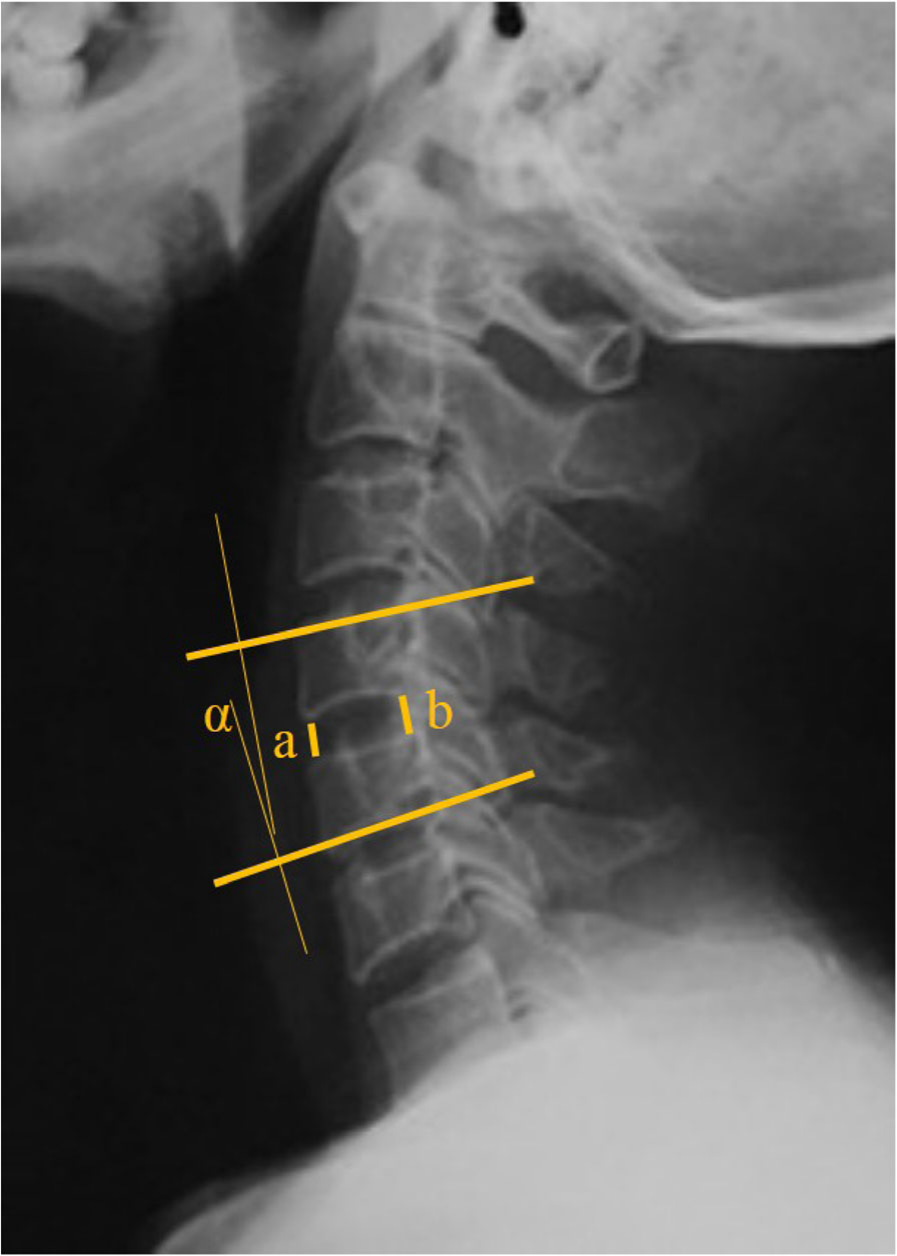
The functional spinal unit angle (*α*) was examined on lateral radiographs and was formed by *lines* drawn at the superior end plate of the cephalad vertebral body and at the inferior end plate of the caudal body. Disc height was defined based on the average values of the anterior disc height (*a*) and the posterior disc height (*b*)
